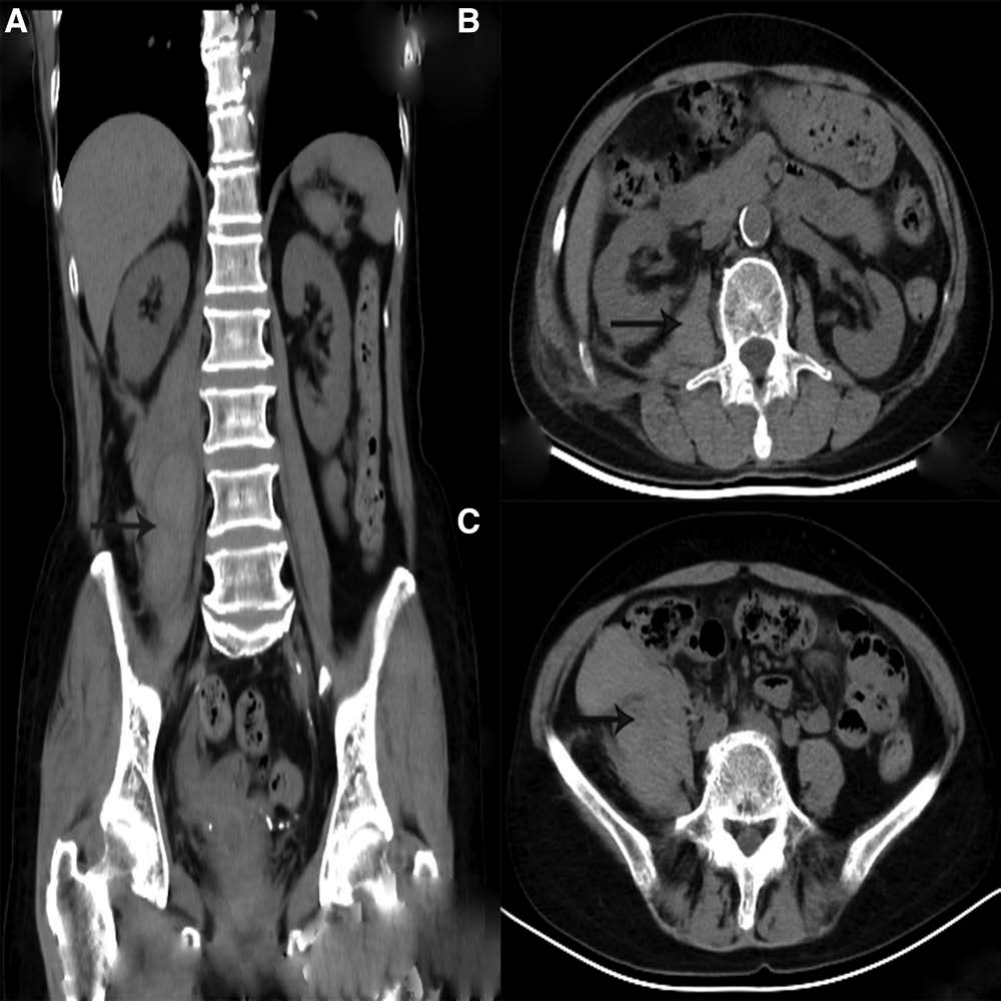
Plain CT image of the abdomen obtained 6 d after the treatment of small needle scalpel. (a) Coronal view; (b) horizontal view at the L2 level; (c) horizontal view at the L5 level. CT = computed tomography.
